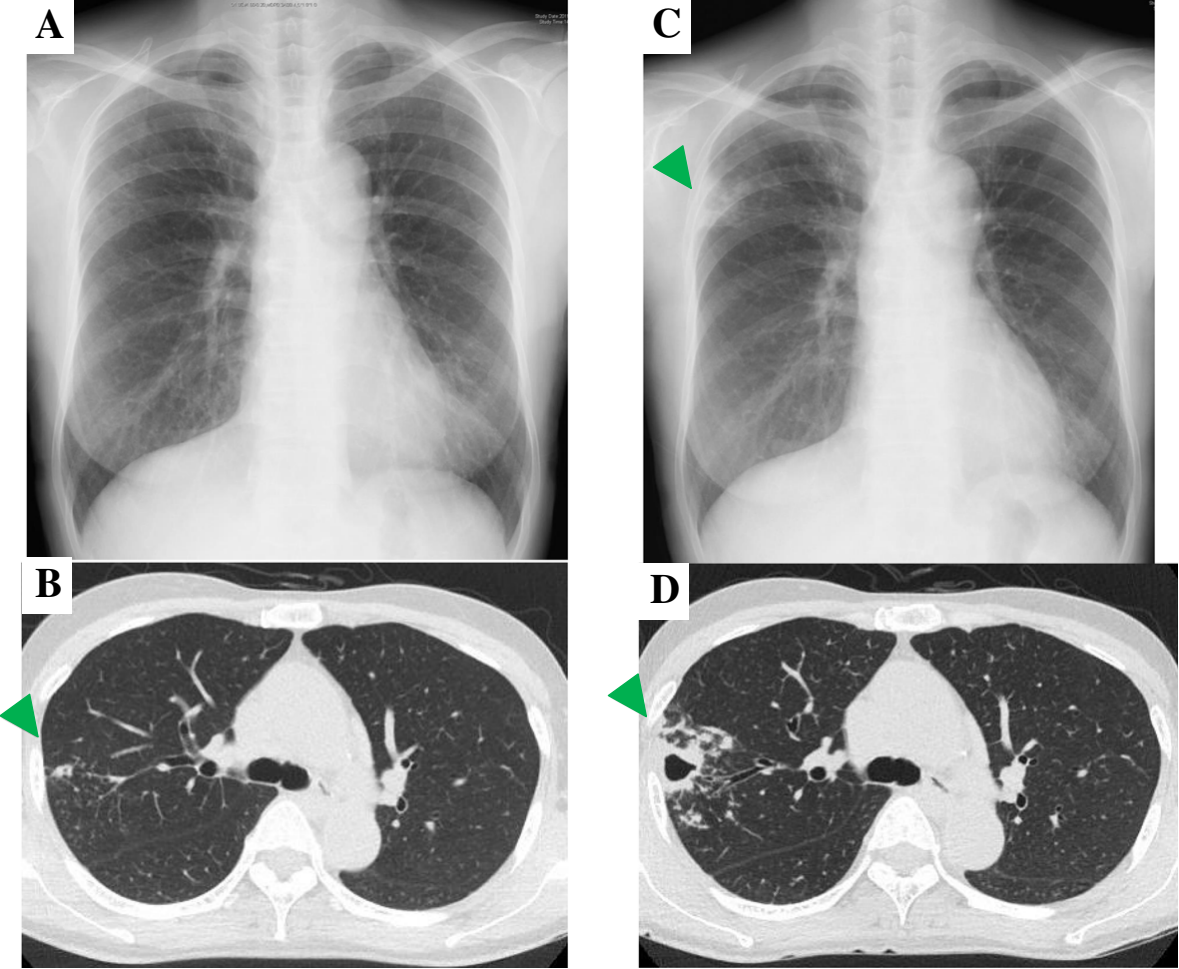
Serial changes on chest X-ray and chest computed tomography findings. a Chest X-ray taken immediately before starting tocilizumab (TCZ), showing subtle nodular infiltrates. b CT scan taken immediately before starting TCZ, showing a small nodular shadow in the right upper lobe (*arrowhead*). c Chest X-ray taken when the patient developed hemoptysis 2 years after starting TCZ, showing infiltration and cavity formation in the right upper lung field (*arrowhead*). d CT scan taken when the patient developed hemoptysis 2 years after starting TCZ, showing consolidation, cavity formation, bronchiectasis, and centrilobular nodules in the right upper lobe (*arrowhead*)
